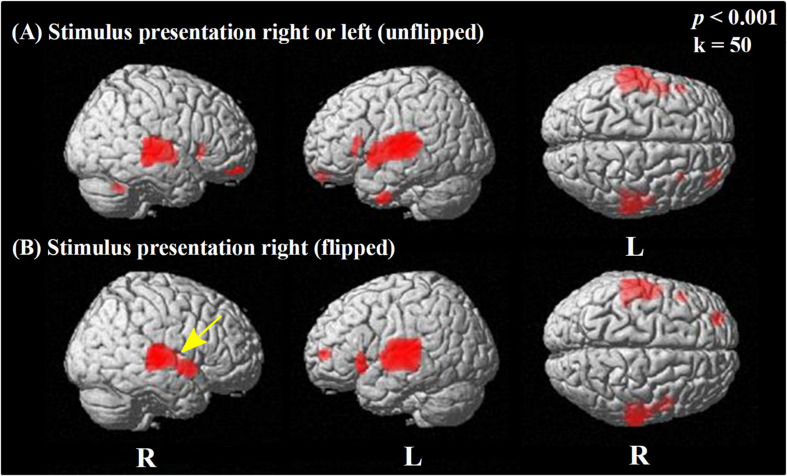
Statistical parametric maps (SPMs) reflecting relative increases of perfusion due to performing the speech condition in comparison to the rest condition. SPMs are overlaid to a surface rendered MRI data set in the Montreal Neurological Institute (MNI) space. There are some areas displayed more transparent than others, which refers to their distance to the projection surface. (A) The results obtained using the original data without left/right flipping are displayed. (B) To explore for potential effects of the side of stimulation, each data set with left-sided stimulation was flipped in the median sagittal plane, while data sets with right-sided presentations remained unflipped. This resulted uniformly in data sets with stimulation from the “right” side in relation to the images for analysis. For both comparisons, the contrast speech condition > rest condition is displayed. Strong significant perfusion increases due to the task are similarly visible in (A,B), showing bilateral activation in the superior and middle temporal cortices and the inferior prefrontal cortex. Note the minor difference between unflipped and flipped images, with a lack of perfusion increase in the ipsilateral (right) primary auditory cortex (BA 41; yellow arrow) for the flipped in contrast to the unflipped image (see also Supplementary Table A.2).
